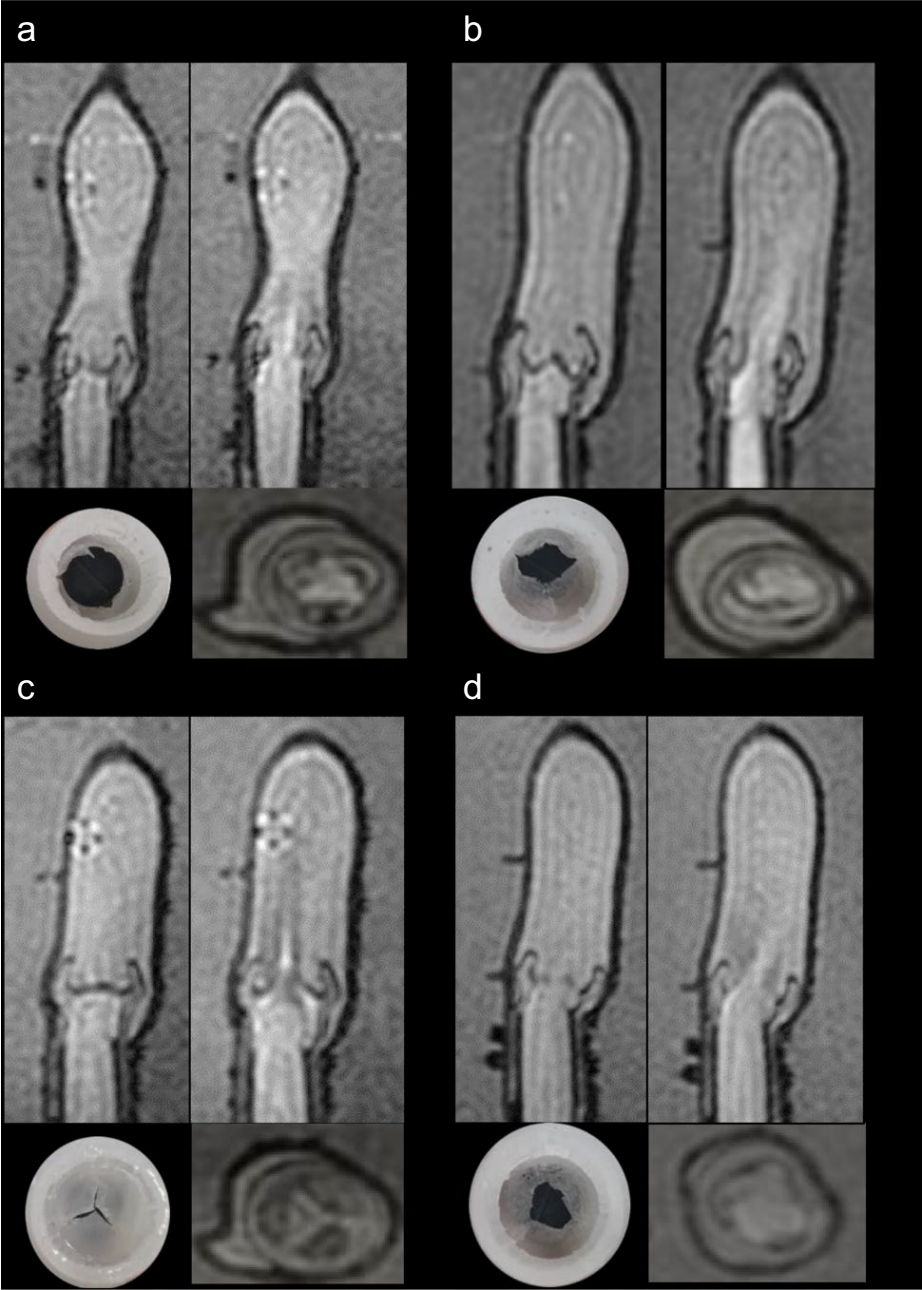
Images of the valves in circuit 2. In each sub-panel, the respective valve can be observed imaged by MRI in closed (left upper) and then open formation (right upper), en face (right lower) and finally the physical valve model (left lower). Four different valves are observed normal (a), bicuspid (b), calcific (c), rheumatic (d)
